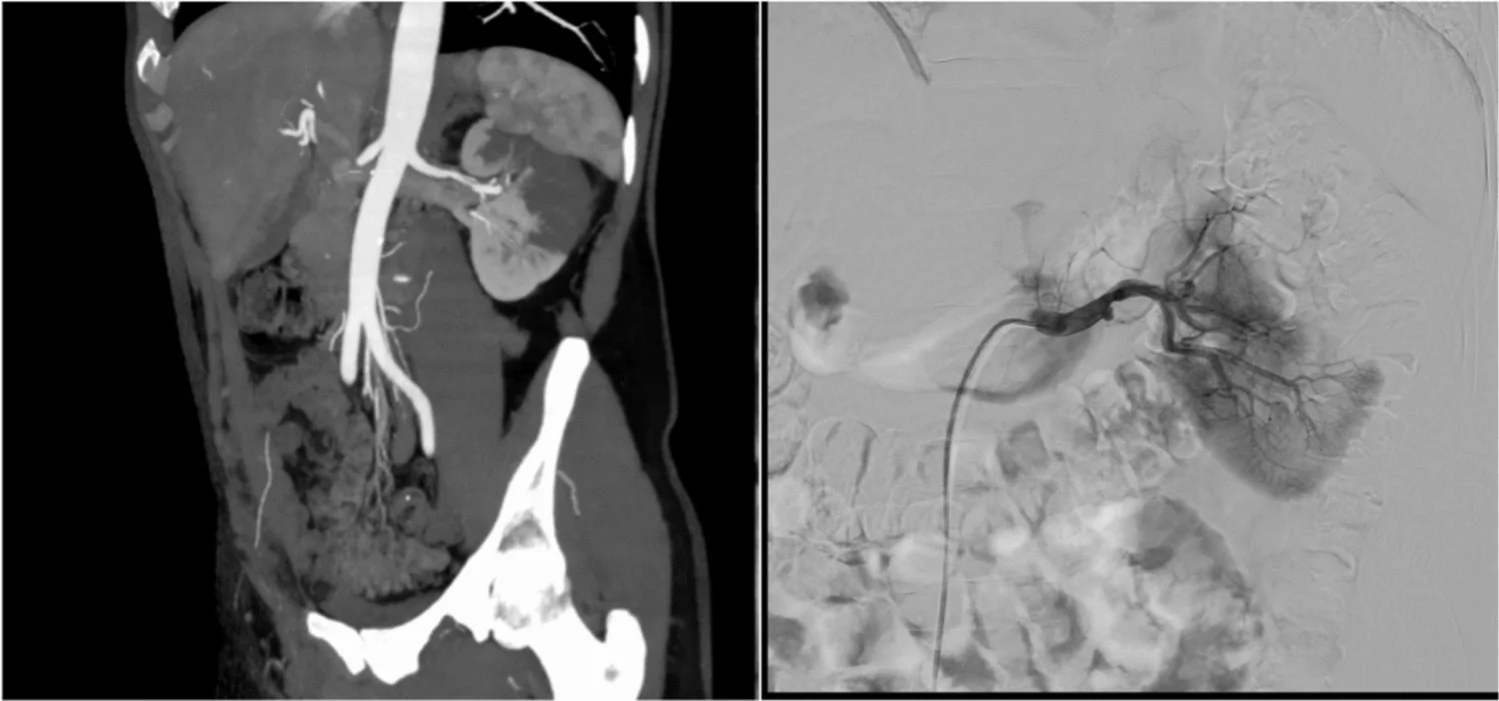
On the left: contrast enhancement defect in the mid-to-upper third of the left kidney, with an infarct-like appearance in the mid-third on CT scan. On the right: angiography showing left renal artery of reduced caliber with trombotic deposition
